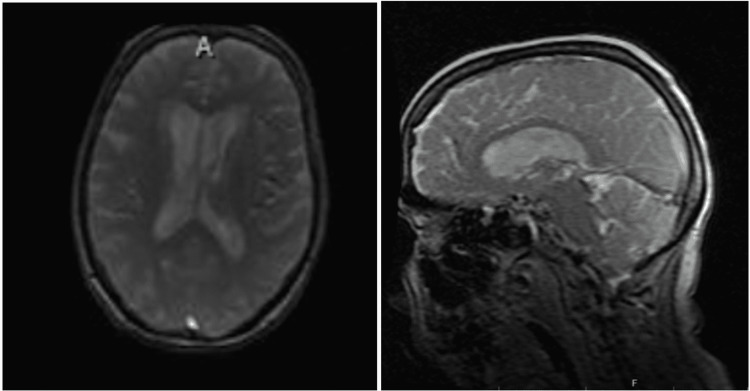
MRI of the brain on February 17, 2022 Restricted diffusion is noted in the medial aspect of the right cerebellar hemisphere likely representing an acute infarct. Additional acute slit-like infarcts are noted in the bilateral cerebellar hemispheres. There is a prominent motion artifact, but there appears to be restricted diffusion at the gray-white junction throughout the bilateral cerebral hemispheres, likely representing acute infarcts.
